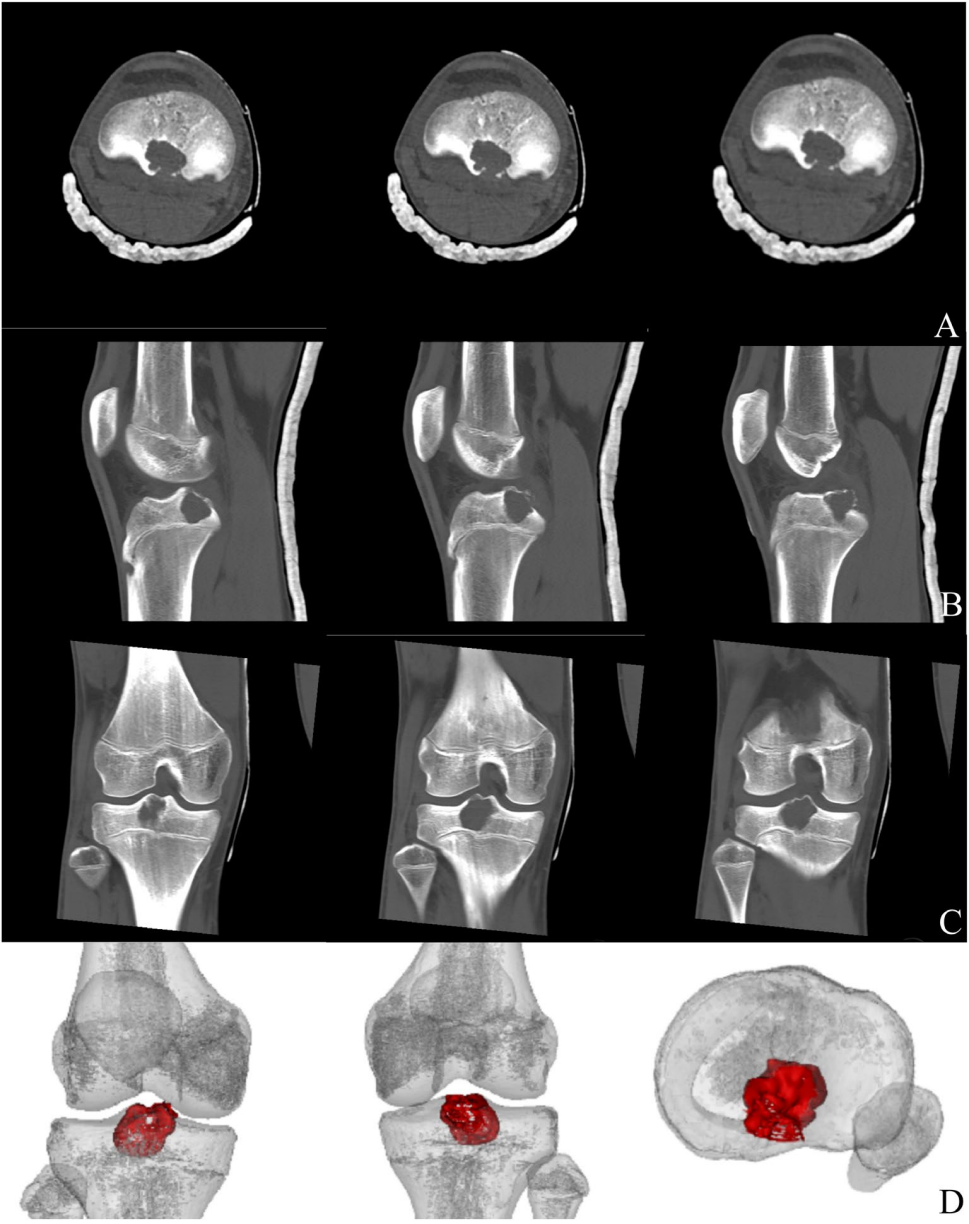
CT scans and three‐dimensional reconstruction showing osteolytic lesion in proximal tibial physis of right knee: axial view (A); sagittal view (B); coronal view (C); and three‐dimensional reconstruction of the lesion (D).
